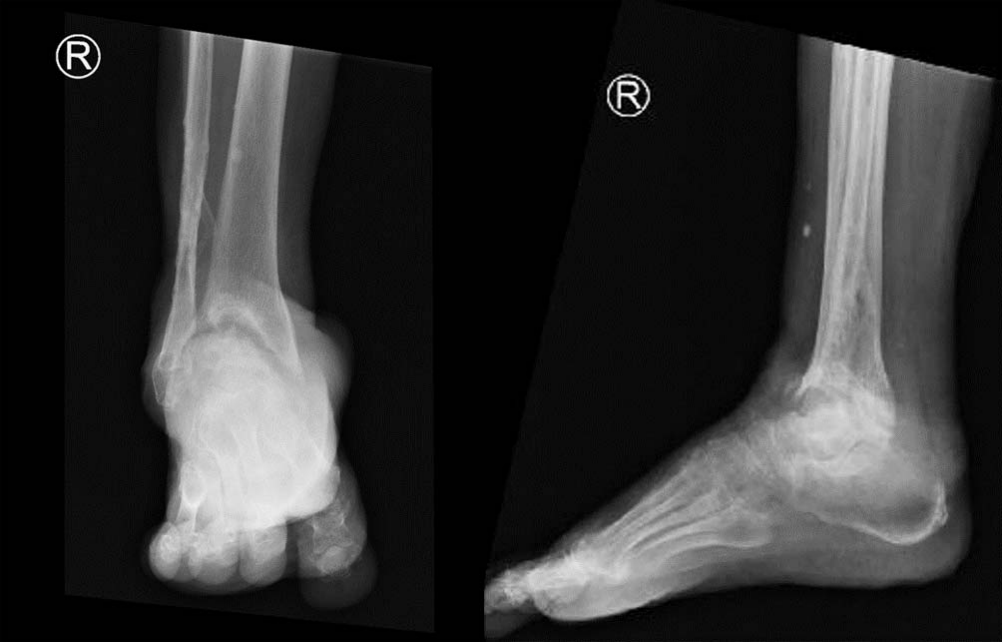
Ankle radiograph showing ankle osteoarthritis with irregular surface that will hamper the bony apposition.
